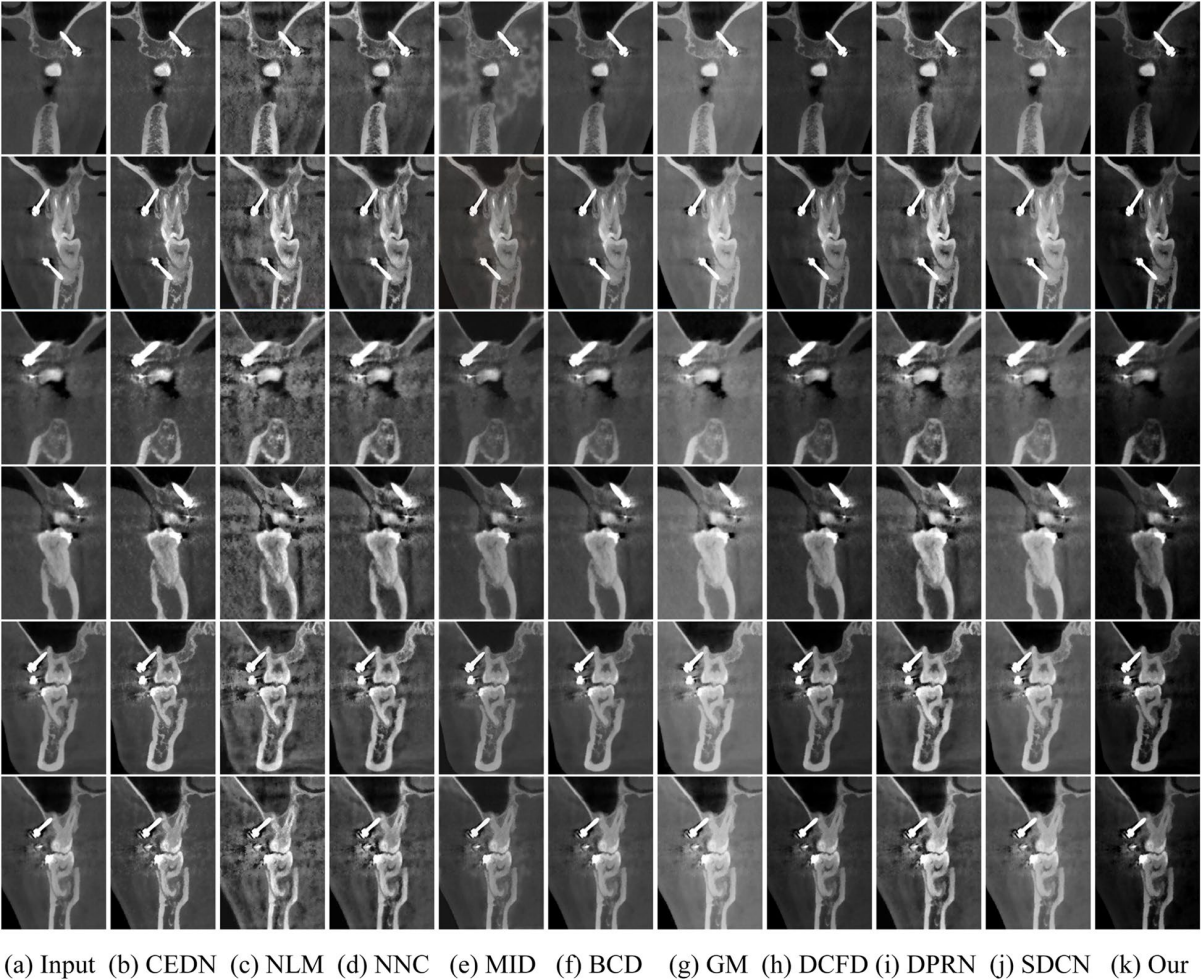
Comparative results of qualitative evaluation of different algorithms in Test 2 dataset. Here we show the enhancement effect of different algorithms on oral CBCT images
